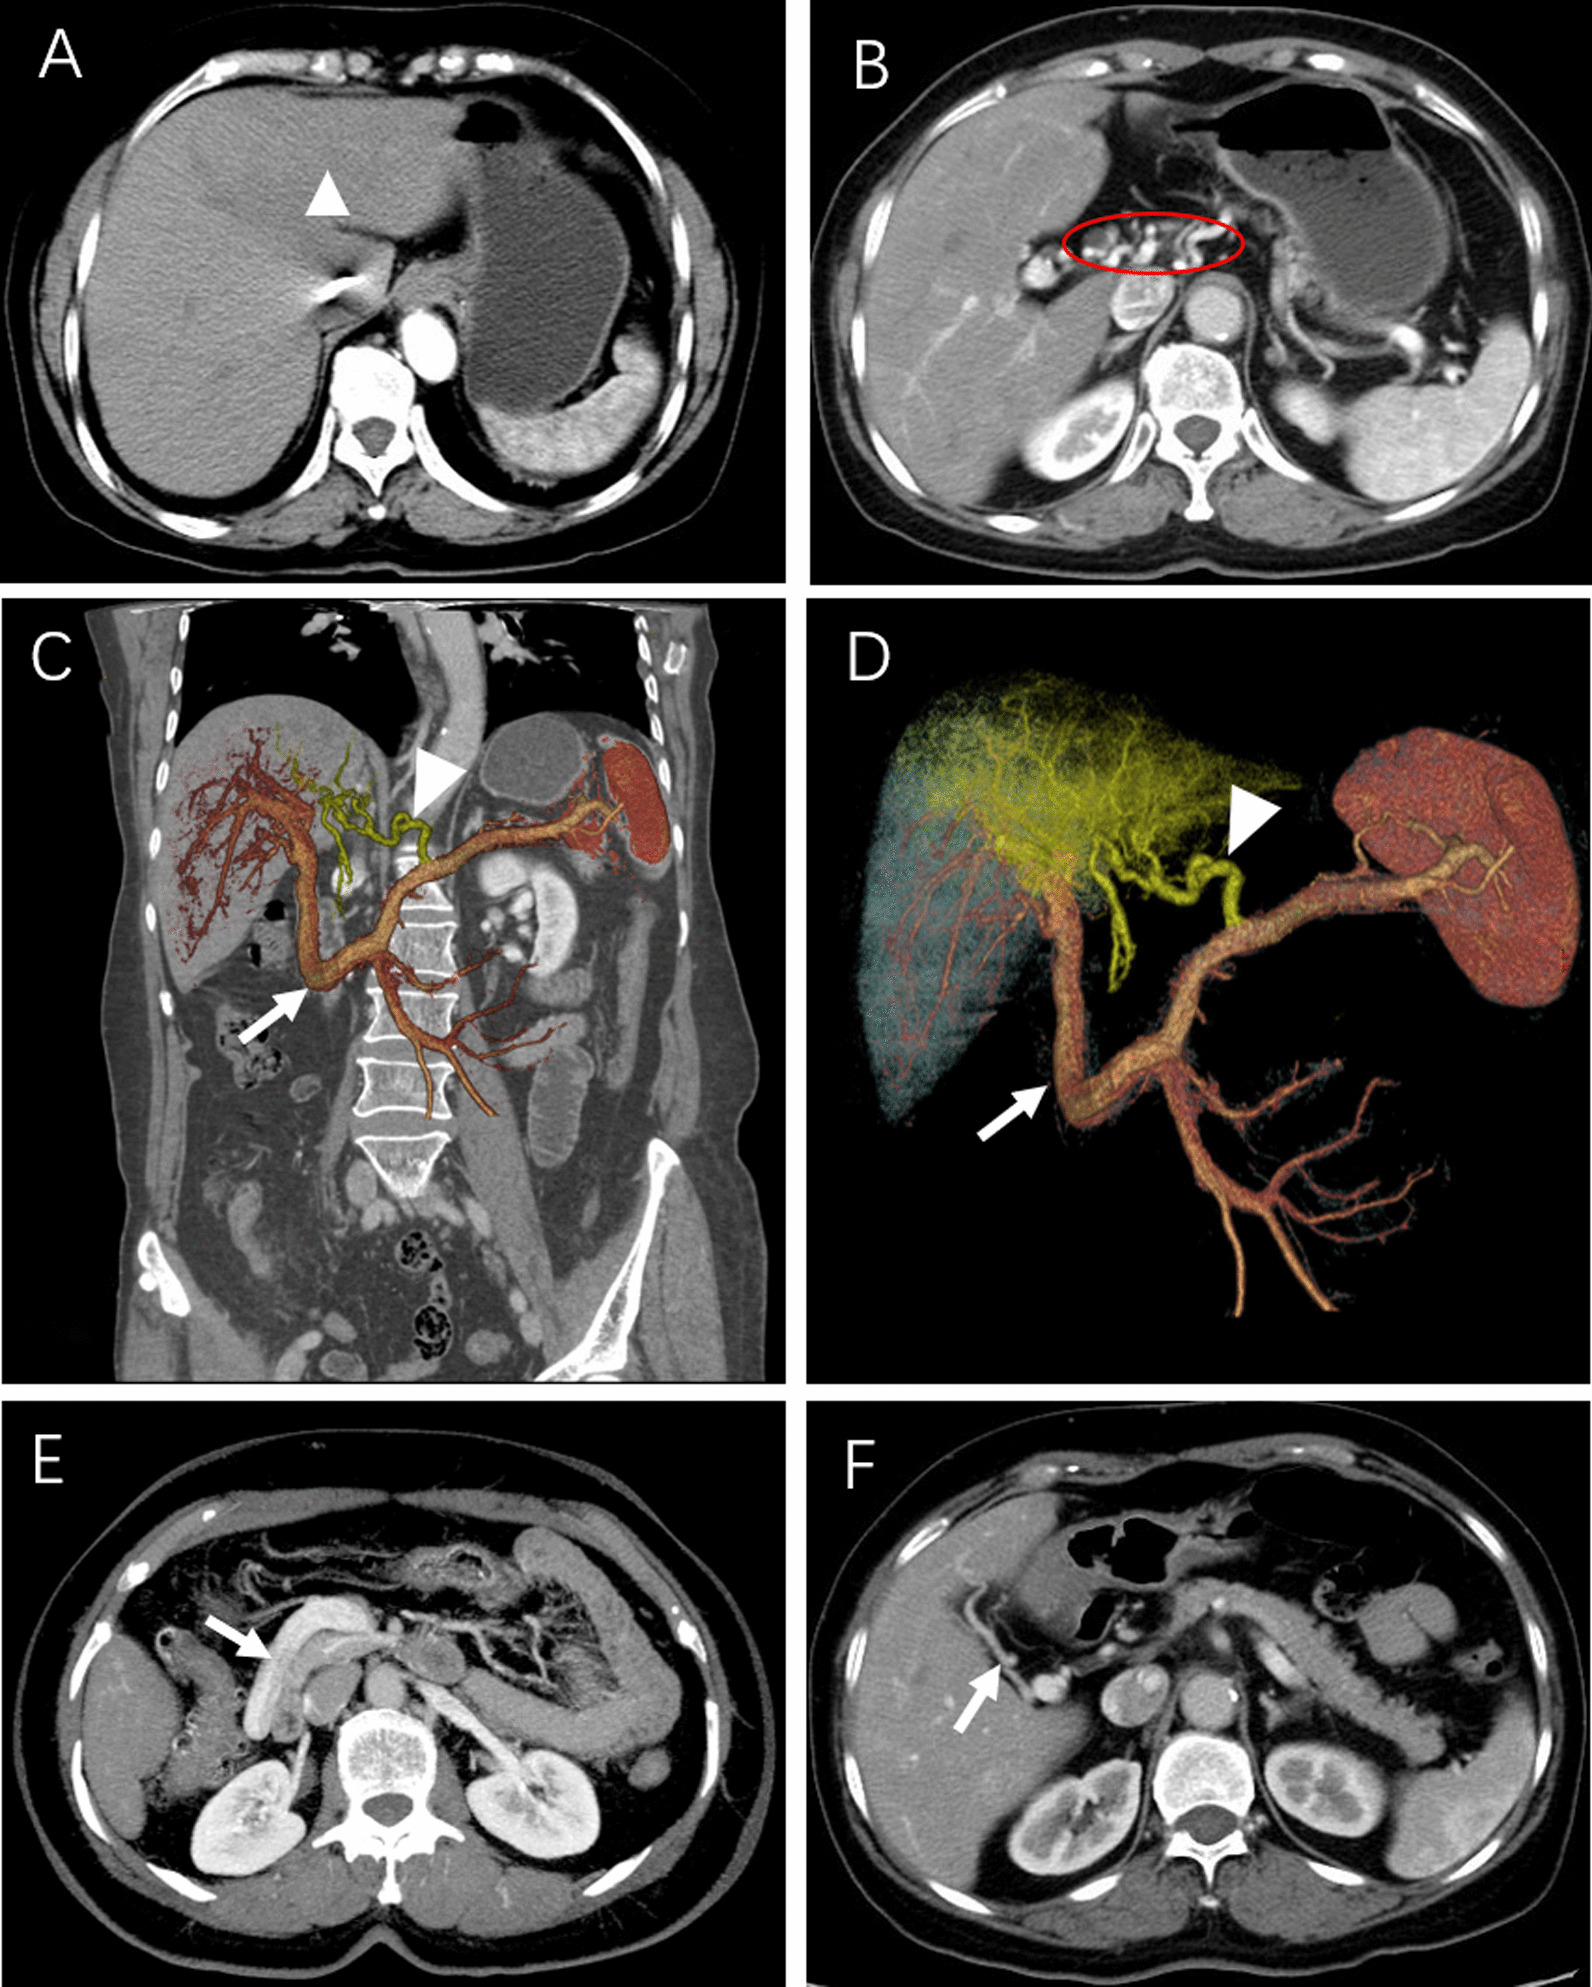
Post-contrast enhanced CT scans of the patient’s abdomen. a Transient perfusion abnormal region at the left lobe of liver (arrowhead). b Tortuous vessels mimic cavernous transformation of the PV (red circle). c–e The VR and MIP images showed SMV and SV join to form a preduodenal portal vein (PV-1) (arrow), and another portal vein (PV-2) (arrowhead) derives from the SV. f The PV-1 directly divided one branch supplied segment IV (arrow)
